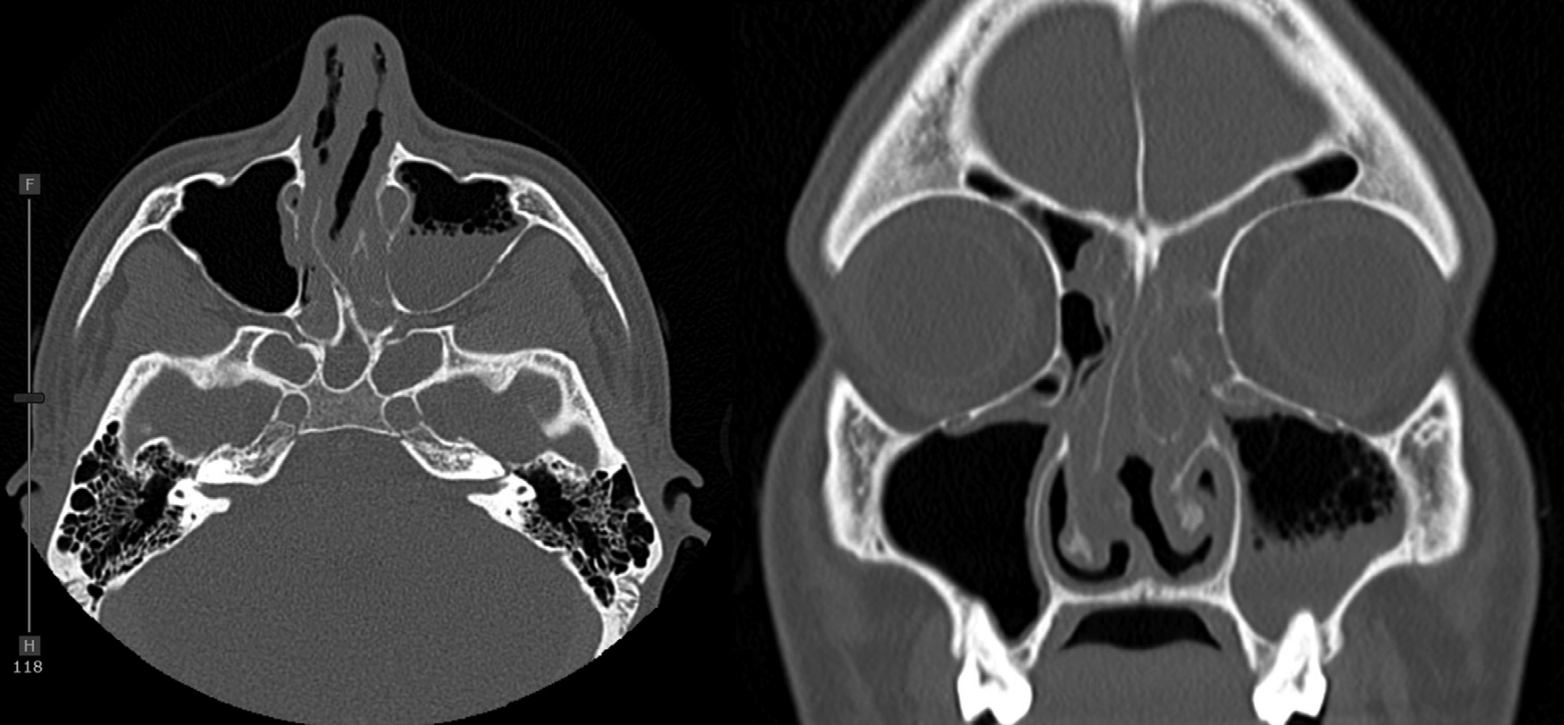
CT sinus protocol showed complete opacification of left frontal and left sphenoid sinus. Frothy secretions with air-fluid level in the left maxillary sinus. Moderate mucosal thickening of the right maxillary sinus and left greater than right anterior and posterior ethmoid sinuses.
